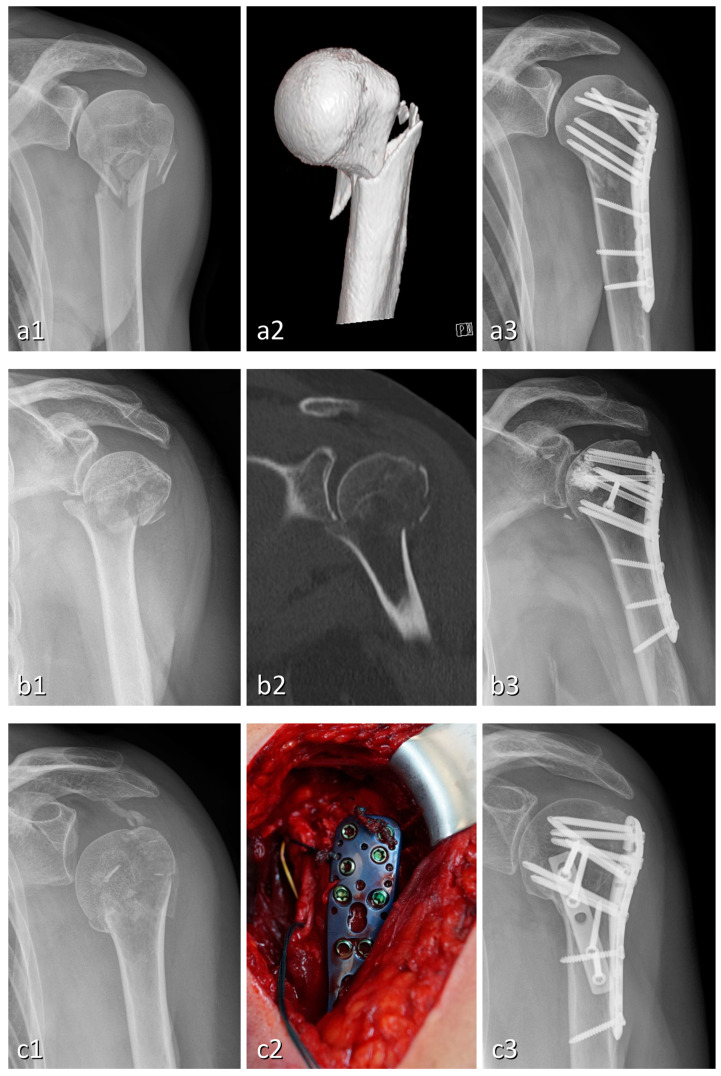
Radiographs of three cases of four-part proximal humeral fractures treated with different variants of locking plate fixation: PHILOS locking compression plate (a), plating with a combination of PHILOS locking compression plate, cement augmentation and additional screw (b), and double plating with one-third tubular plate (c). Each case is presented with pre- and post-operative X-ray (a1, b1, c1 and a3, b3, c3, respectively) and an exemplary step in the diagnostic/therapeutic process: volume rendering technique (a2), CT with coronal re-construction (b2), surgery with plate implantation via deltoidopectoral approach (c2).
